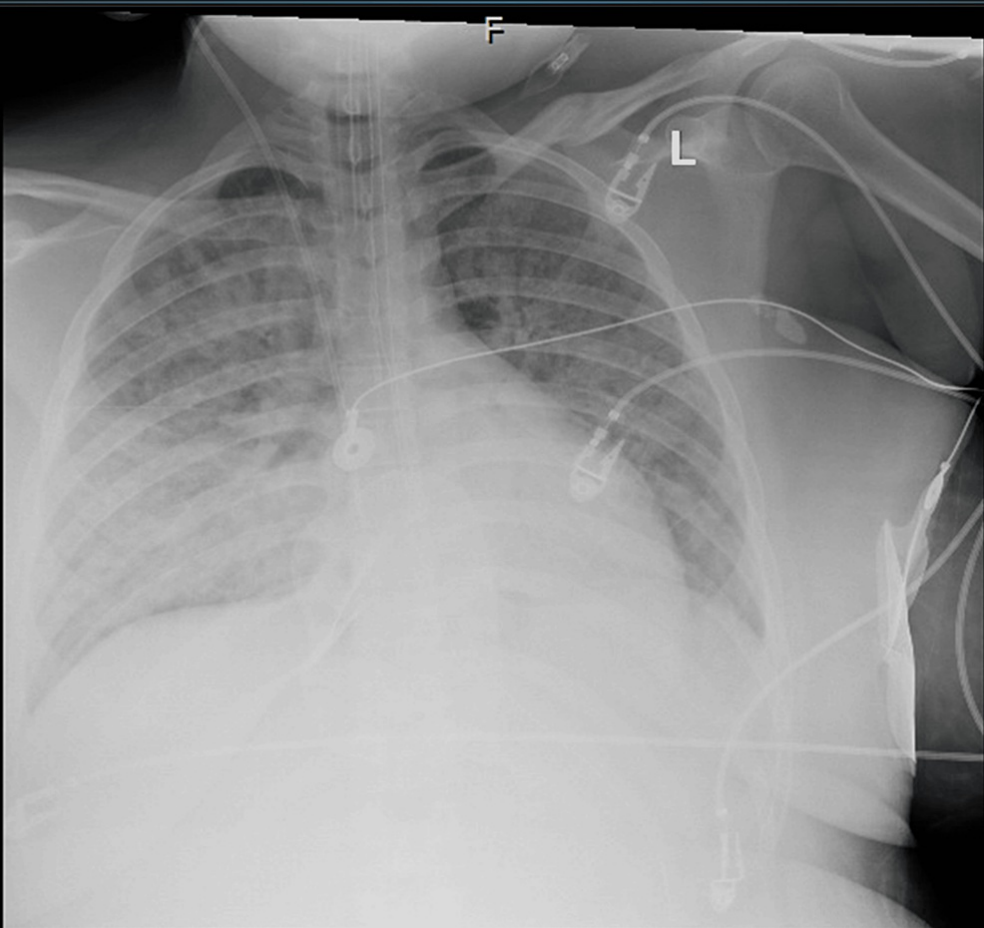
Chest X-ray showing extensive bilateral infiltrates representative of pulmonary edema
